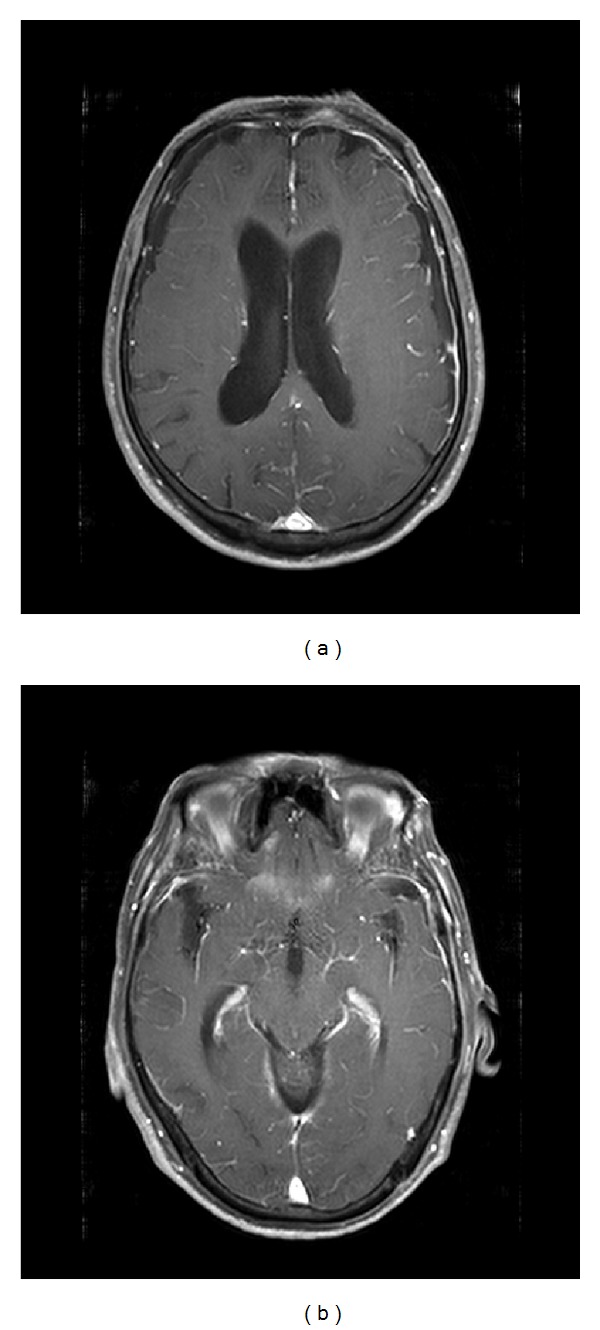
Brain magnetic resonance imaging with contrast showed leptomeningeal enhancement without parenchymal lesion.
